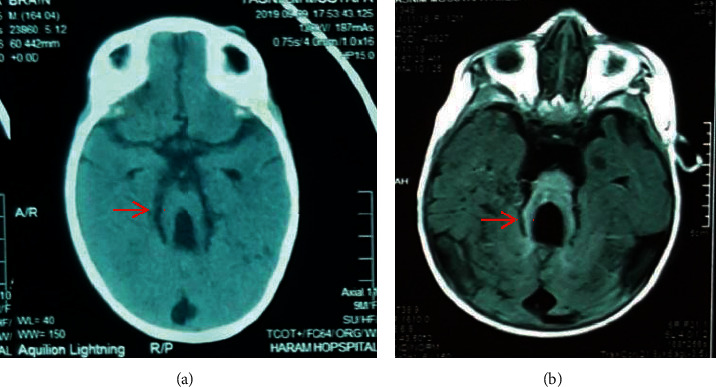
Axial brain imaging. Brain CT scan (a) and brain MRI (b) showing a molar tooth sign at the level of the midbrain (red arrows).
